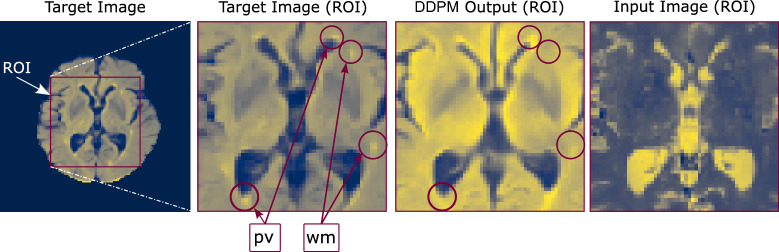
T2 to FLAIR transformation from the OASIS test dataset using the DDPM model. From left to right: the target FLAIR image, the ROI in the target image with white matter lesions (wm) and periventricular hyper-intense regions (pv), the ROI in the transformed image, and the ROI in the input T2 image.
